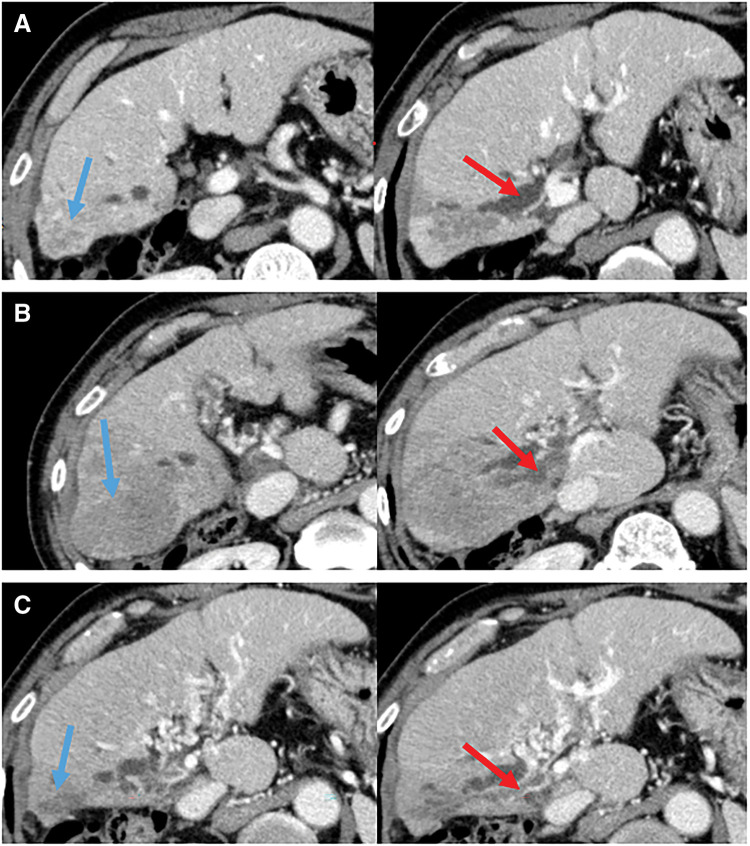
CT findings during treatment course. (A) Tumor in S6 of the liver at the time of recurrence diagnosis. (B) Progressive disease after 2 courses of Atezo+Bev, according to RECIST. (C) Partial response after 3 courses of durvalumab plus tremelimumab, according to RECIST criteria. Blue arrows indicate the lesion in liver S6. Red arrows indicate tumor thrombus in the right posterior branch of the portal vein. Atezo+Bev, atezolizumab plus bevacizumab; RECIST, Response Evaluation Criteria In Solid Tumors; S6, segment 6
